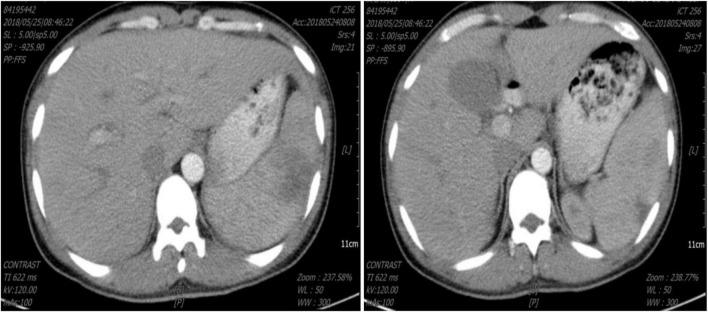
Abdominal computed tomography showed splenic infarction.
